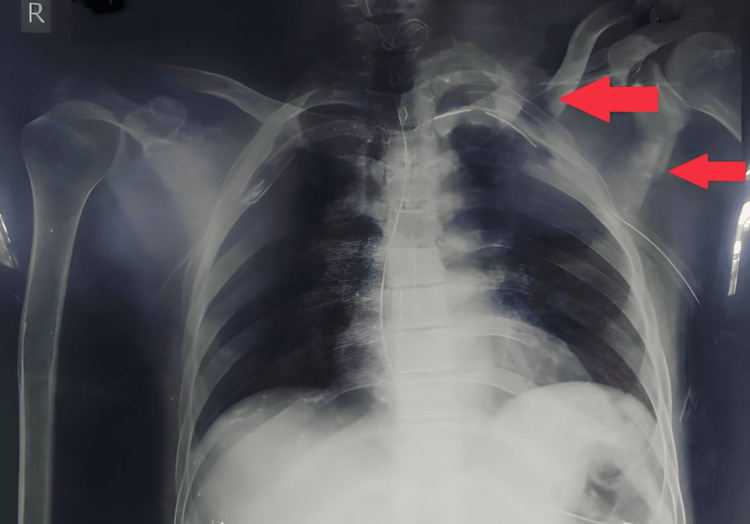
Preoperative X-rays of fracture of the middle third of the clavicle and scapula.
